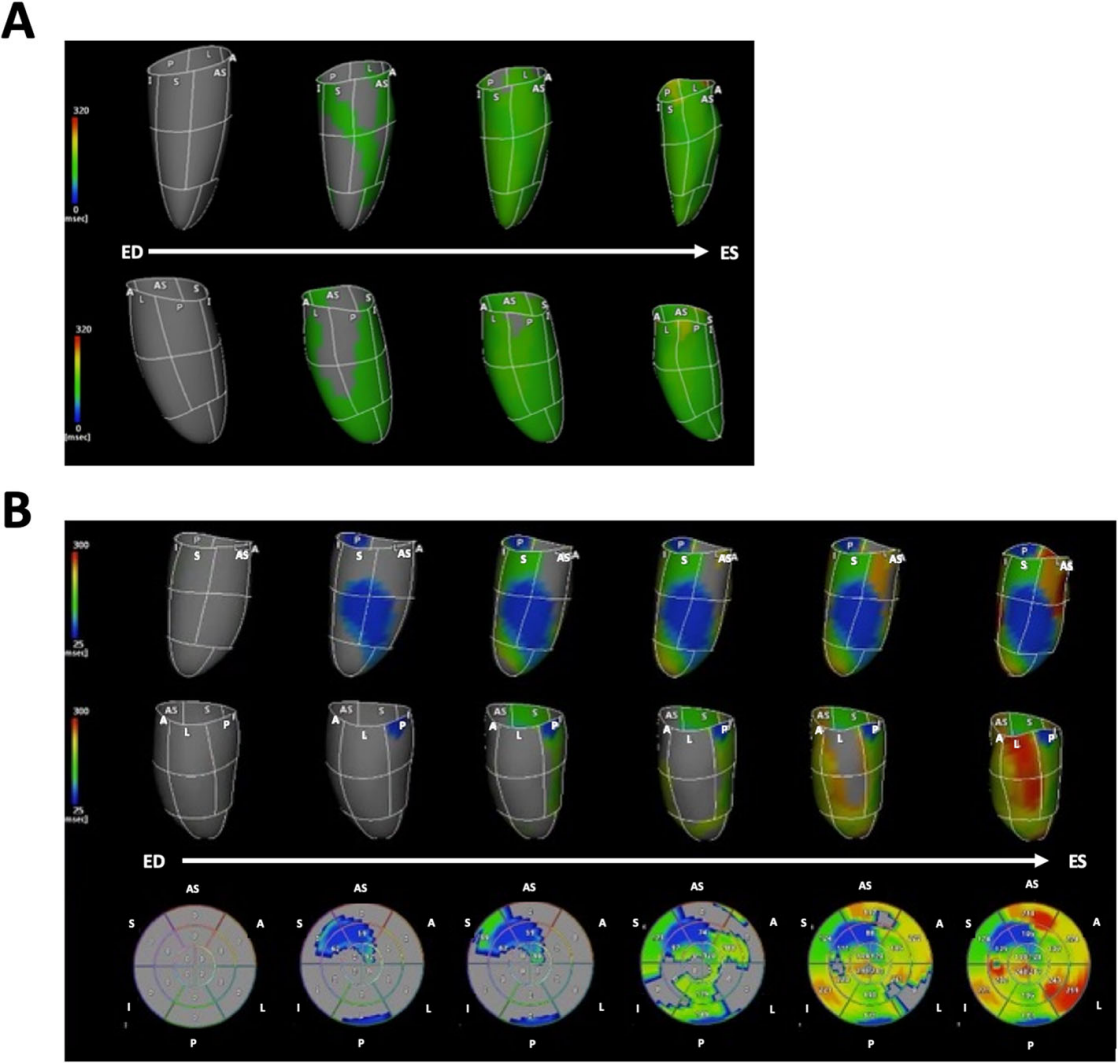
Activation mapping. a: Propagation images in a normal subject by activation imaging with 3D speckle tracking echocardiography. The upper panel shows propagation of myocardial contraction viewed from the left ventricular (LV) septal side from end-diastole (ED) to end-systole (ES), and the lower panel shows it viewed from the LV free wall. The color bar refers to a color-coded time scale that corresponds to the timing of the onset of regional contractions. In this case, the range is set from 0 ms (dark blue) to 320 ms (red) via green and yellow. The onset of myocardial contraction in the entire left ventricle is almost synchronized, reflecting light green colored area spreading rapidly. b: Propagation images in a patient with left bundle branch block. The upper panel shows propagation of LV myocardial contraction viewed from the septal side, and the middle panel shows it viewed from the LV free wall. The lower panel is a bull’s eye map displaying the spread of contraction. The upper panel shows early contraction in the septum colored in blue, which is earlier than septal contraction timing in normal subjects, and corresponds to “septal flash” during the pre-ejection period. The propagation of septal contraction is blocked at the anterior wall, but propagates toward the apex. The delayed propagation from the apex to the lateral wall is shown in a red-colored area in the basal to mid lateral wall, characterizing U-shaped propagation. A, anterior wall; AS, anterior septum; I, inferior wall; L, lateral wall; P, posterior wall; S, septal wall
